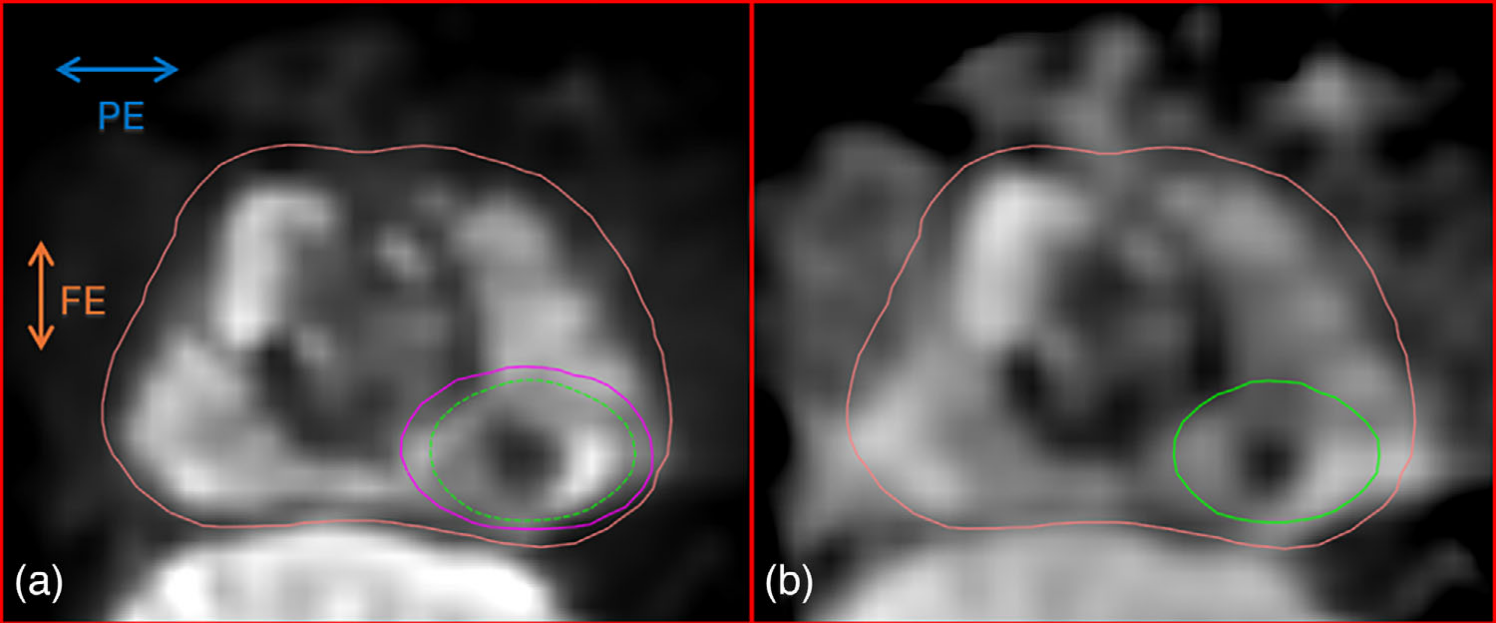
The measured artifact extensions around a GFM on an in‐vivo prostate, delineated by the outer pink ROI. (a) Diffusion weighted image (*b* = 0 s/mm^2^). The magenta ROI represents the artifact extension in DWI, while the dashed green ROI indicates the artifact extension of the corresponding ADC map superimposed on DWI. (b) ADC map. The solid green ROI delineates the artifact extension in the ADC map. Note the difference in size between the artifact extension in (a) and (b). All images were acquired using the same parameters as listed in Table 1.
